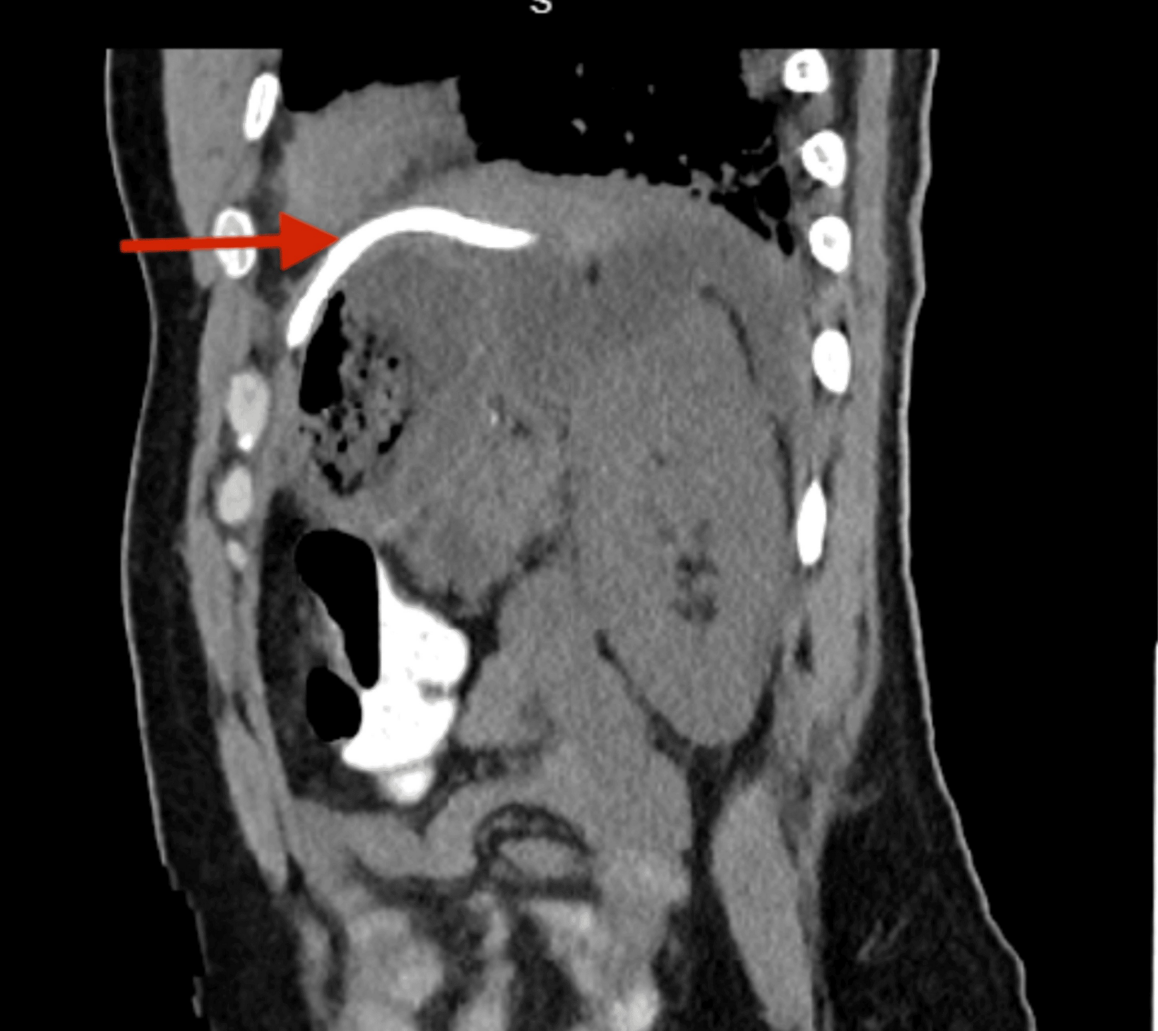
Sagittal view of CT abdomen showing the drain in communication with the collection (red arrow)
